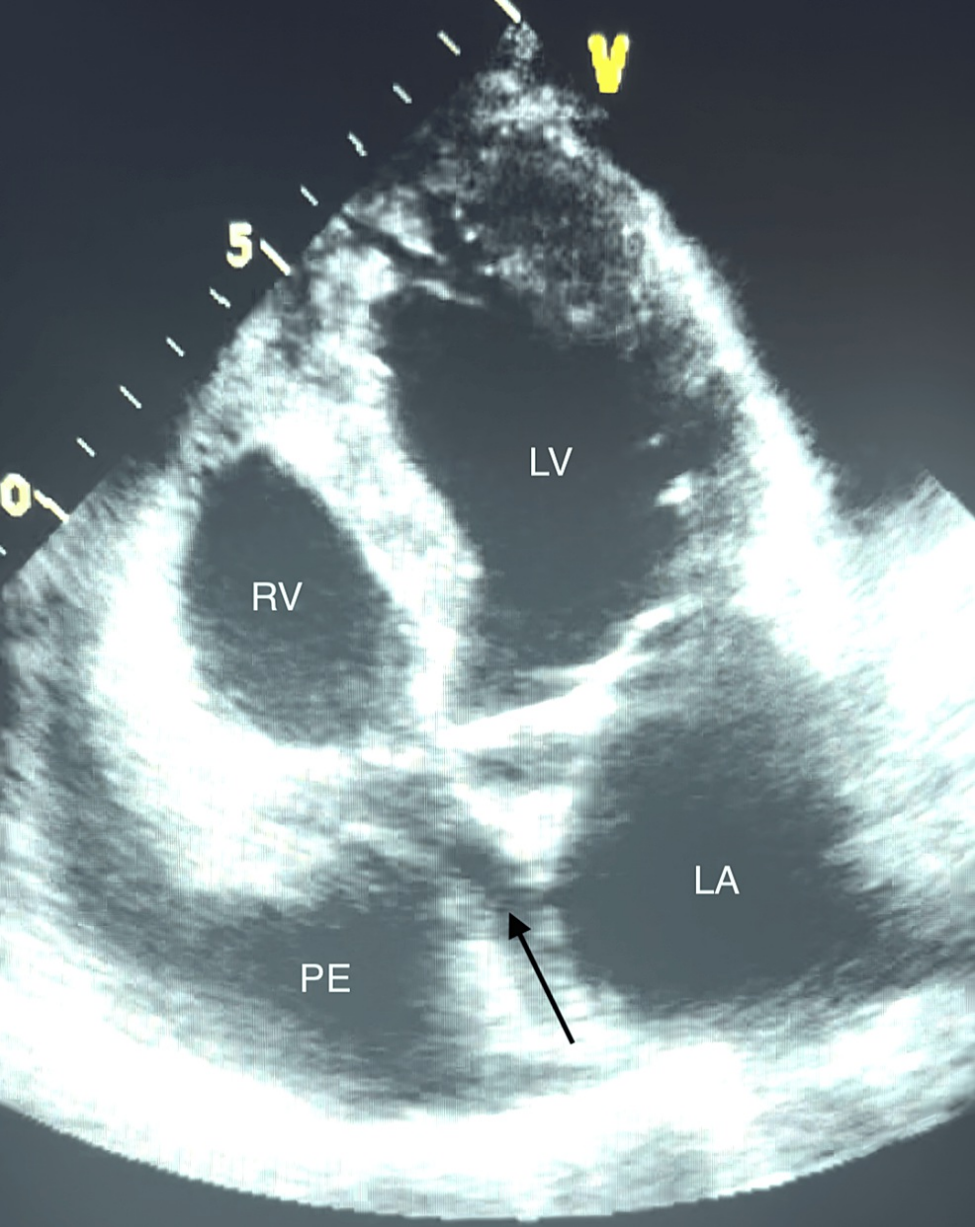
Apical view on TTE revealing pericardial effusion causing right atrial collapse The arrow denotes the collapsed right atrium as a result of circumferential pericardial effusion. LA: left atrium; LV: left ventricle; PE: pericardial effusion; RV: right ventricle; TTE: transthoracic echocardiogram
